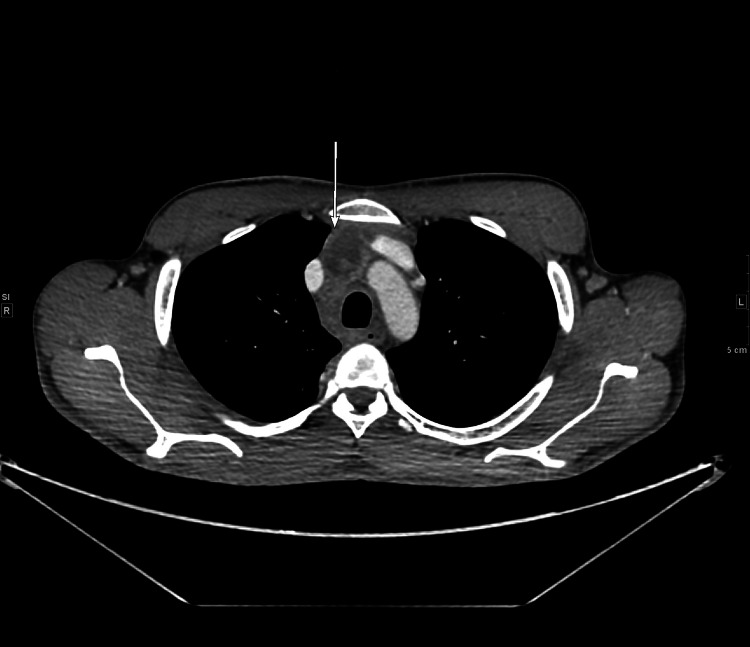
CT scan chest (axial view) after about five months of treatment Newly enlarged lymph node shows the progression of the disease and failure of the previous standard regimen. It is a 25x30 mm prevascular necrotic lymph node.
